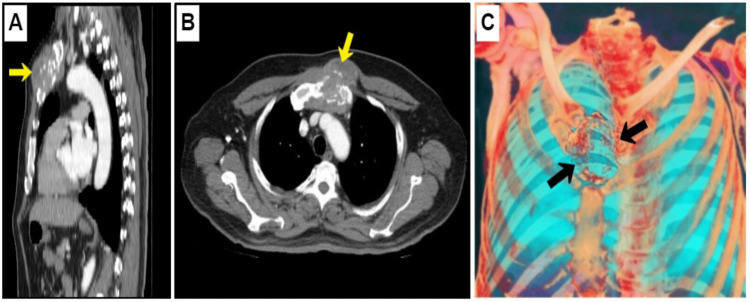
Computed tomography (CT) of the chest. (A) Sagittal view. (B) Cross-sectional view. Contrast-enhanced chest CT: (A-B) shows metachronous bone metastases in the sternum of 5.6 x 5.9 x 7.2 cm (yellow arrows). Three-dimensional CT image: (C) shows erosion and destruction of the sternal manubrium by expansive tumor growth (black arrows).
